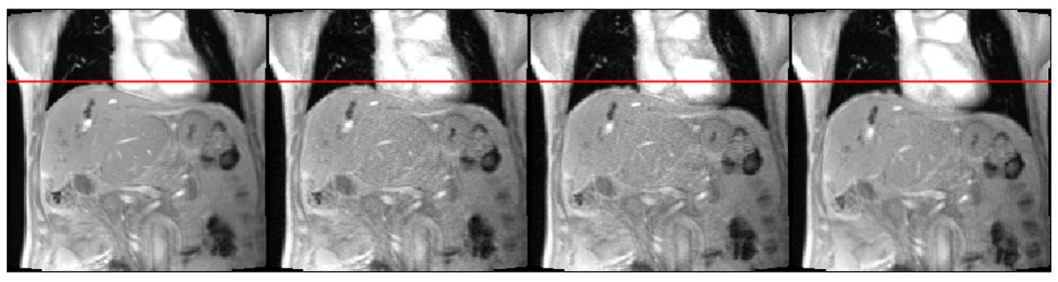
Binned and averaged MRI slices for 4 out of the 10 bins; ranging from end-expiration to end-inspiration.
